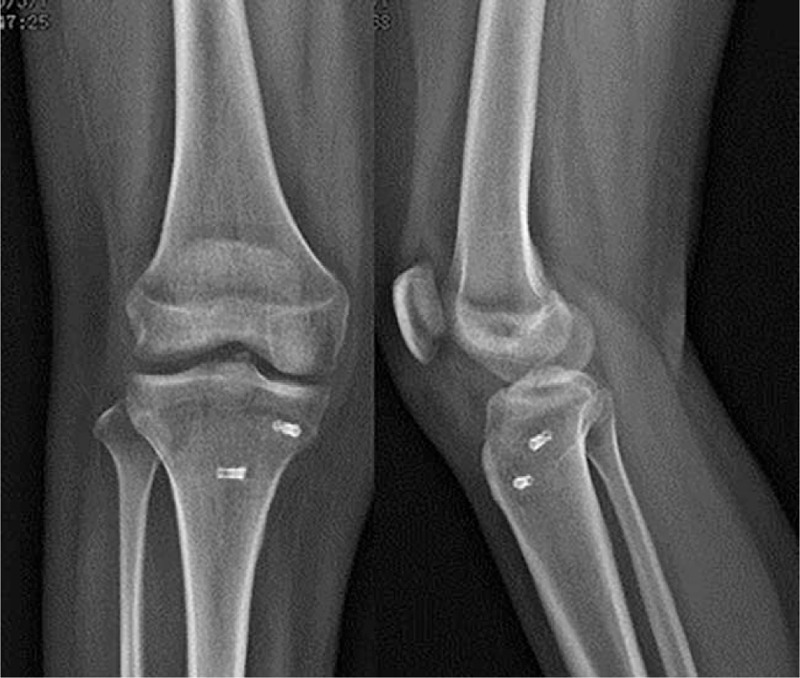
Radiograph showing satisfactory healing of the fracture.
